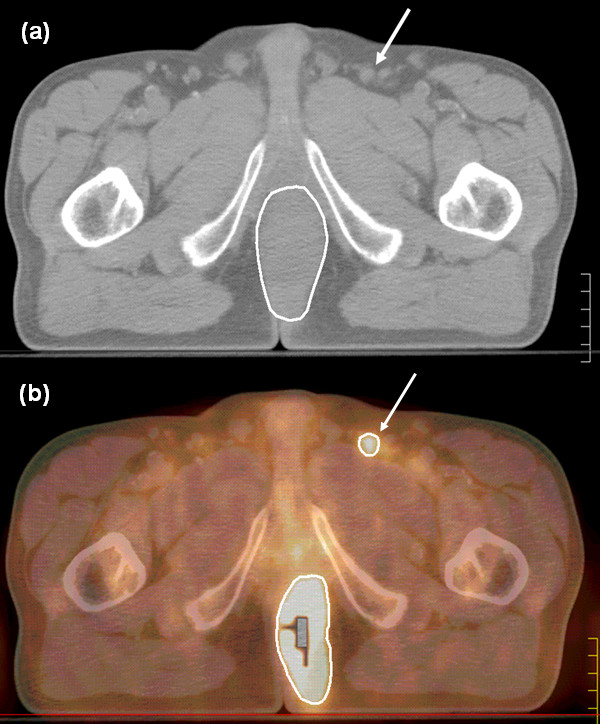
(a -- b) CT (a) and PET/CT (b) in axial view showing a lymph node of about 1 cm in diameter with intense uptake at PET (arrows). Positivity was confirmed by FNAB. Based on these finding, this lymph node was included in the GTV and received a boost of radiation dose.
